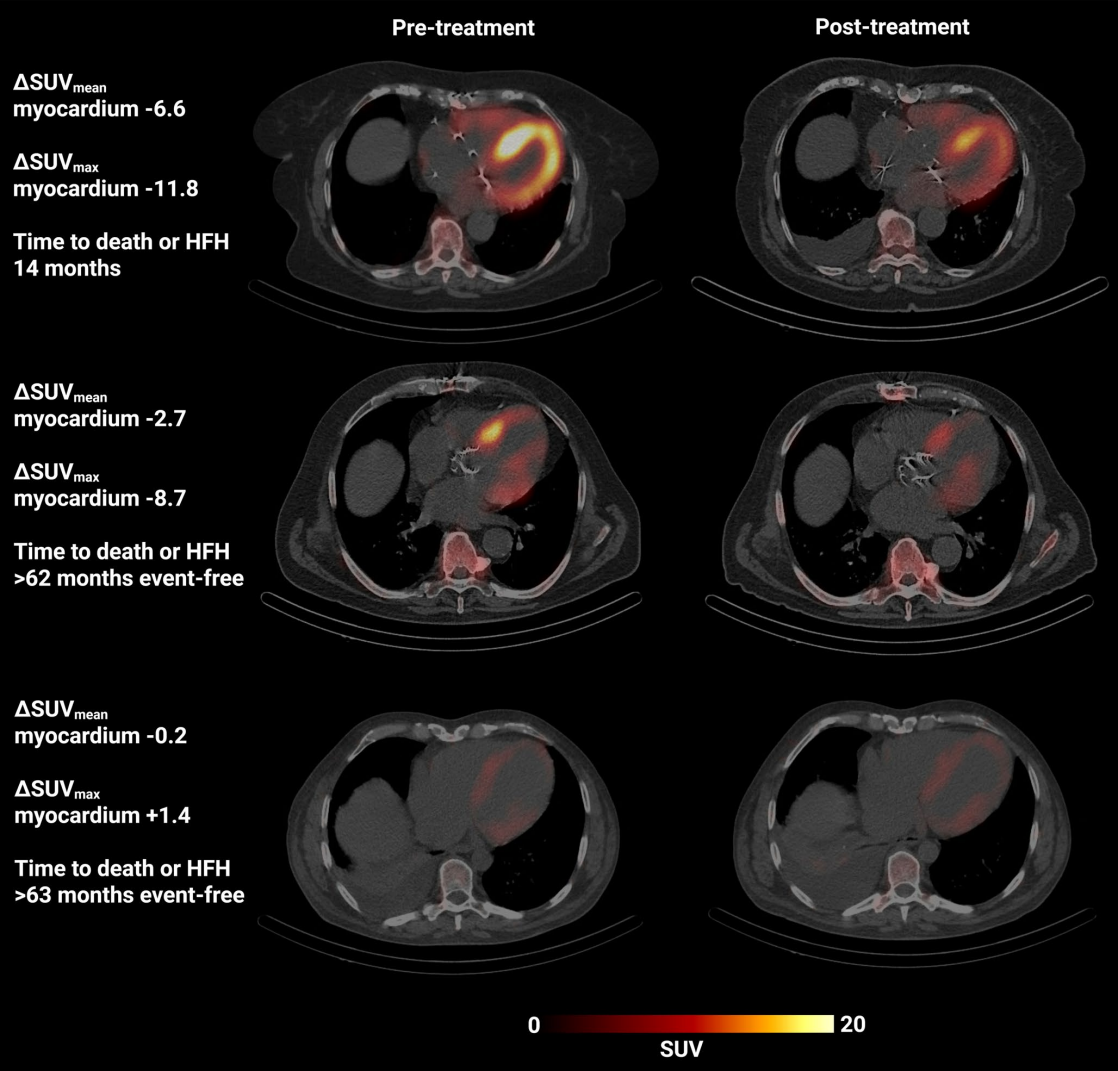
Representative SPECT/CT images of three patients (rows) before and after treatment
